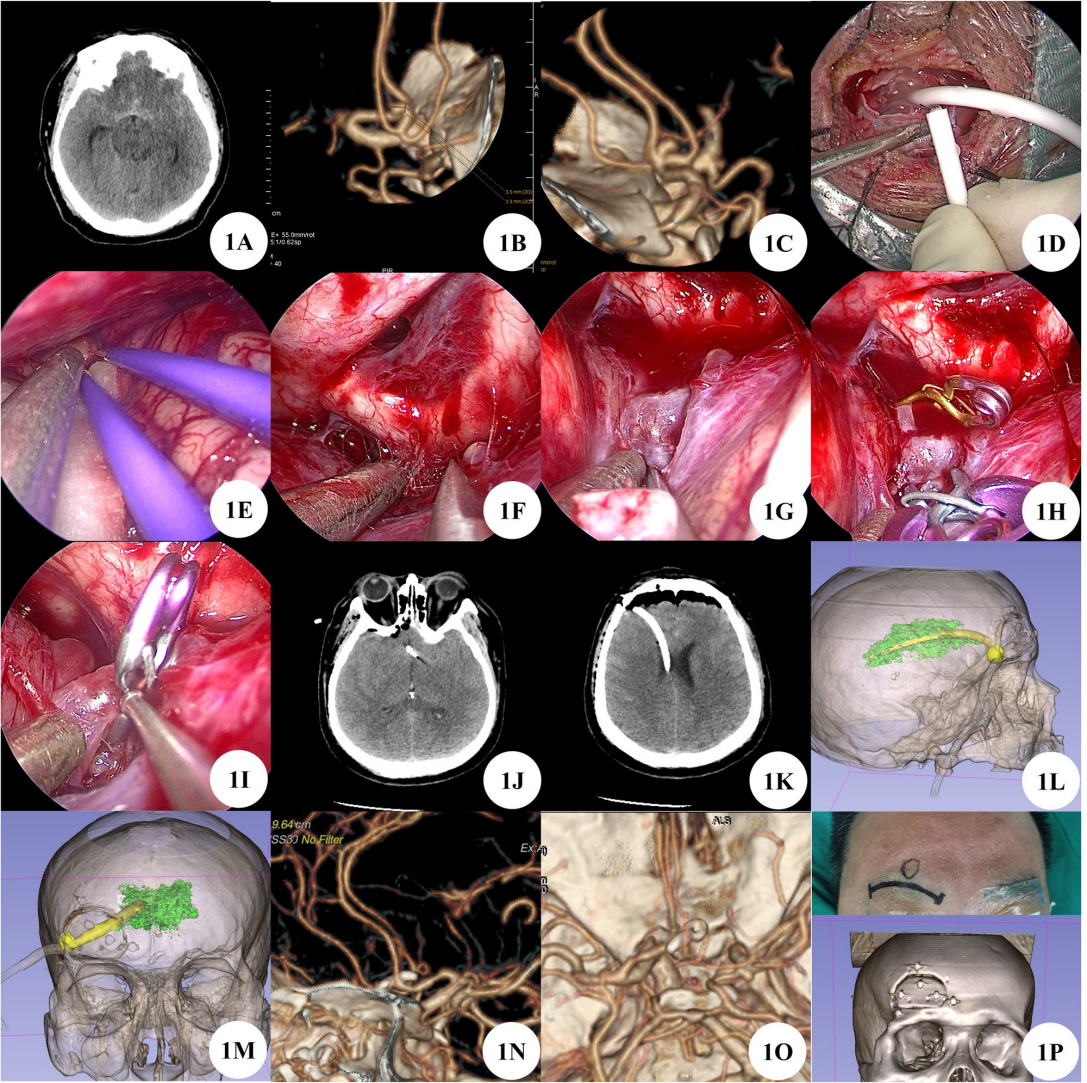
(A) Preoperative CT shows subarachnoid hemorrhage. (B–C) Preoperative CTA suggests anterior communicating artery aneurysm. (D) Intraoperative puncture of the lateral ventricle to release cerebrospinal fluid for decompression. (E–I) Separation and clipping of aneurysms by neuroendoscopy. (J) Postoperative CT shows aneurysm clip. (K) Postoperative CT scan revealed ventricular drainage tube. (L,M) Postoperative 3D Slicer reconstruction of virtual reality images of lateral ventricles and extraventricular drainage tubes. (N,O) Postoperative CTA indicates complete occlusion of the anterior communicating artery aneurysm, with no stenosis in the parent artery and branches. (P) Surgical incision and bone window.
